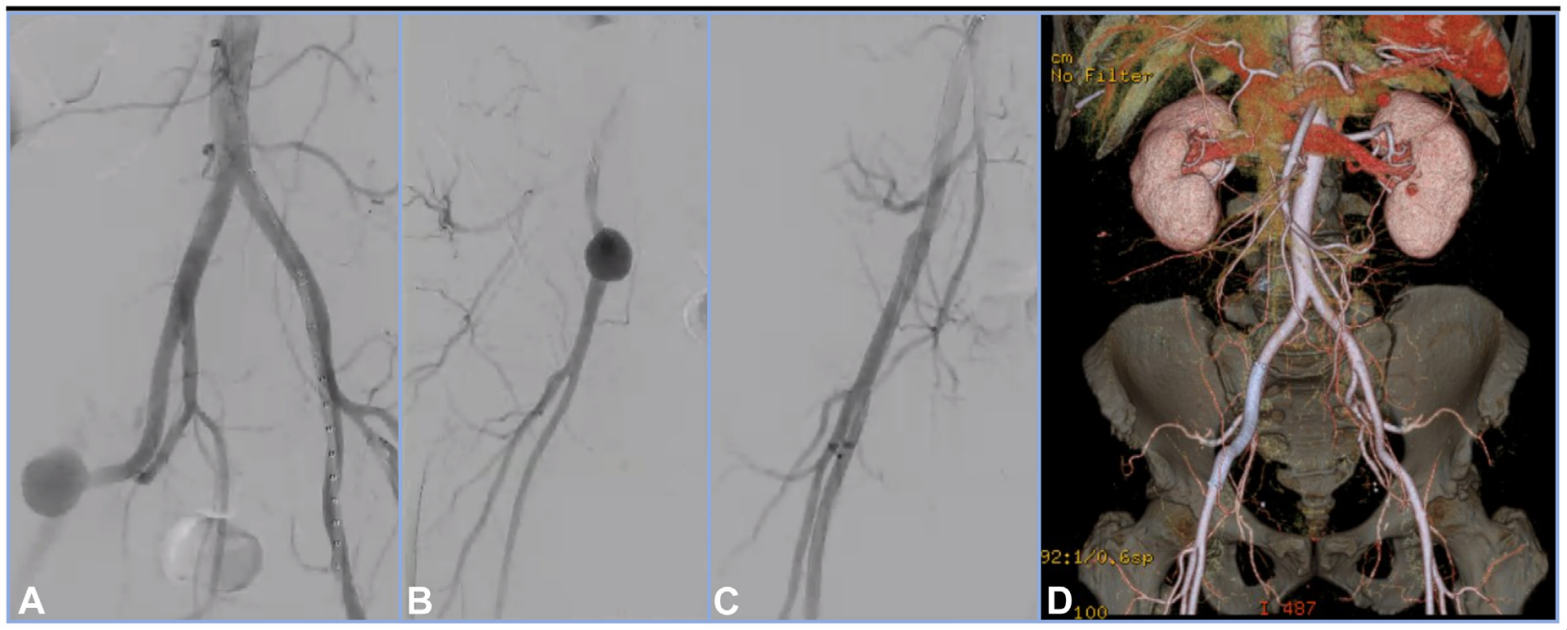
Intraoperative angiography. (A) Preoperative aortogram. (B) Preoperative iliac angiogram. (C) Completion angiogram after stent graft deployment. (D) Reconstructed three-dimensional image of 2-week follow-up computed tomography scan showing completely excluded aneurysm with patent stent graft in suprainguinal right external iliac artery (*EIA*).
